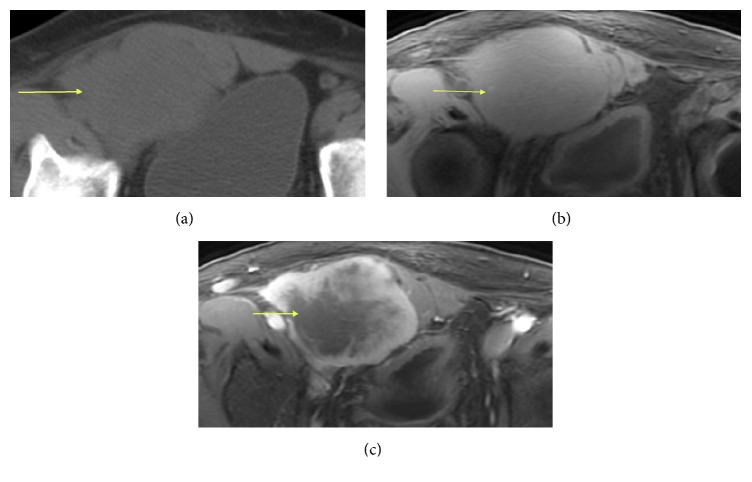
Typical radiological appearance of a 47-year-old man with IMT of the right groin. (a) An isodense, lobulated soft tissue mass in the right groin. (b) T2-weighted MRI showed a homogenous iso-signal mass. (c) Gadolinium-enhanced T1-weighted fat-suppression images showed heterogeneous enhancement (yellow arrow).
